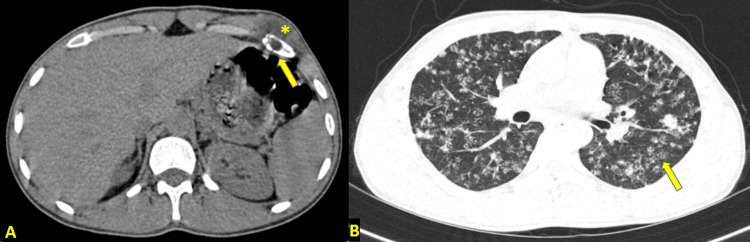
(A) HRCT image of the chest: soft-tissue window showing hypodense collection (marked by an asterisk) in the intramuscular plane of left external oblique muscle and eroded anterior part of the left seventh rib (depicted by arrow). (B) HRCT image of the chest: lung window showing multiple randomly distributed soft-tissue density nodules (depicted by arrow) diffusely distributed in bilateral lung fields, many of them showing a tree-in-bud pattern. HRCT = high-resolution computed tomography
